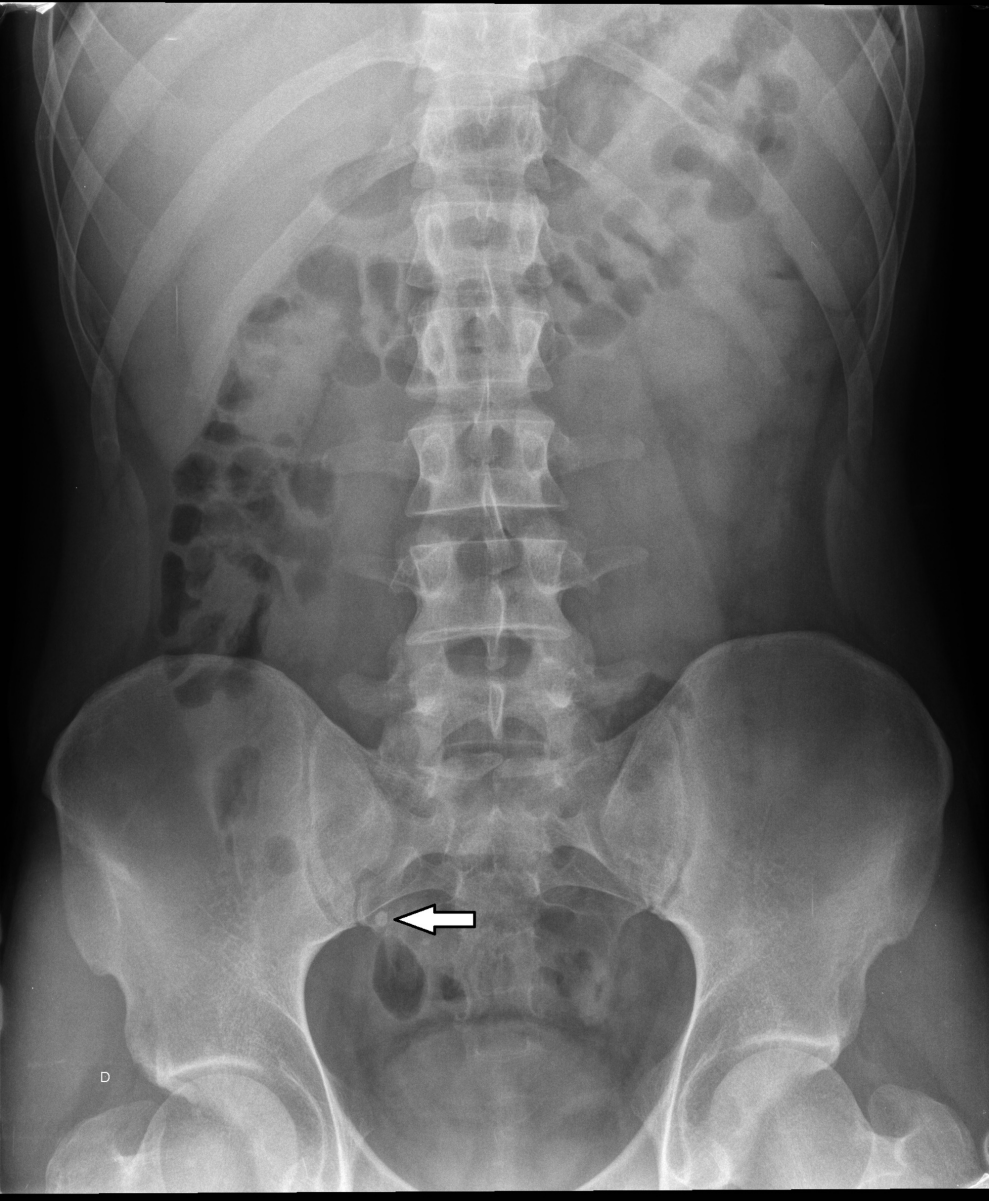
Simple abdominal X-ray. Phebolites are shown overlying the right sacrum (white arrow).
